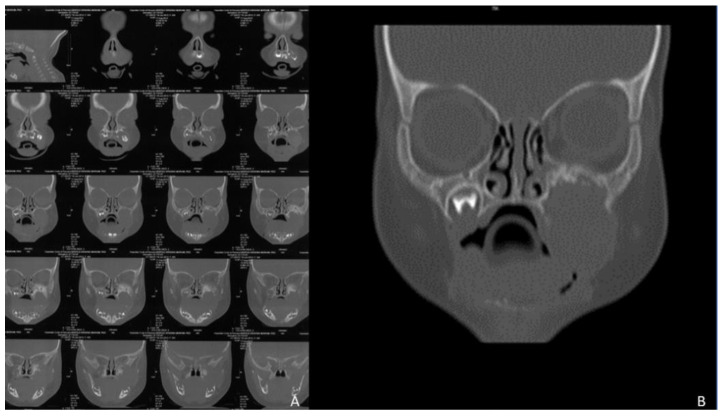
(A,B): Cone-beam of the maxillofacial region at 40 days of age.
